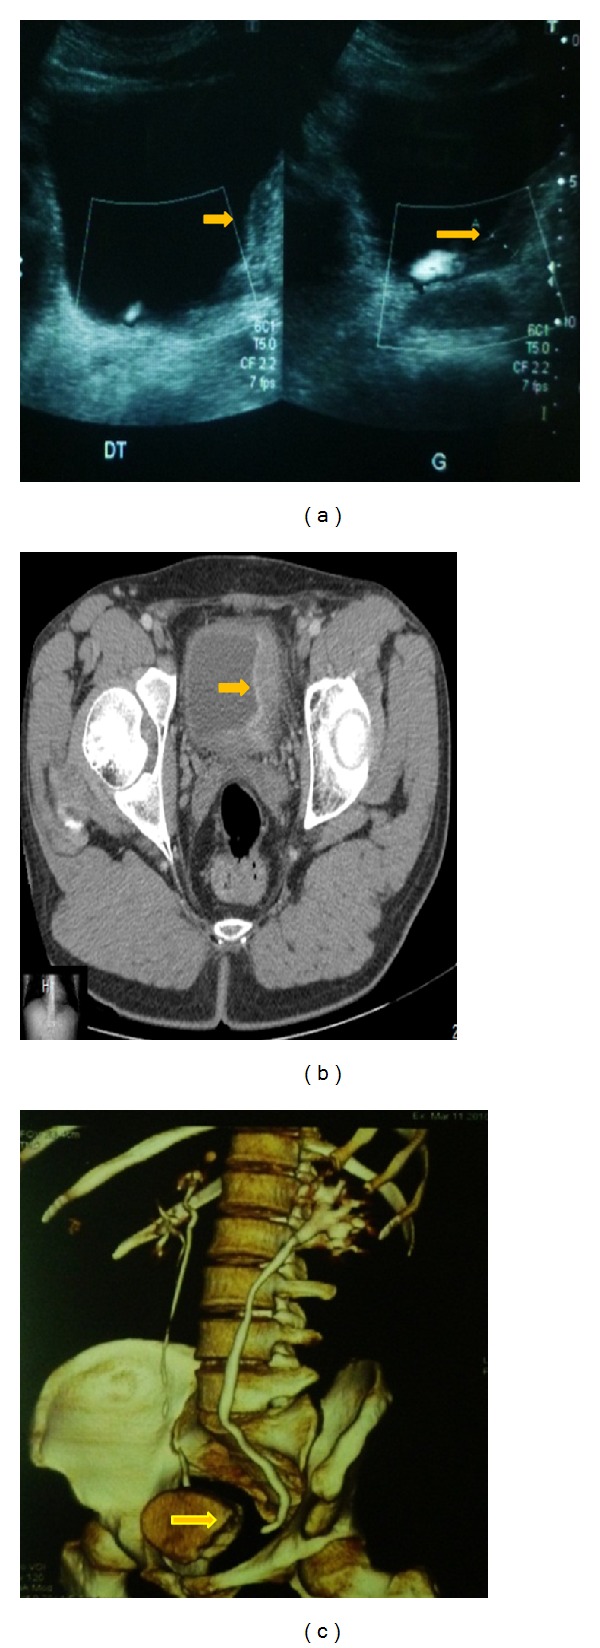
(a) Ultrasound: large mass of the left wall of the urinary bladder. ((b) and (c)) Contrast-enhanced CT scan shows marked and diffuse bladder wall thickening, bladder tumour mainly on the left but extending to the midline anteriorly and beyond the midline posteriorly with moderate dilatation of the upper ureter.
